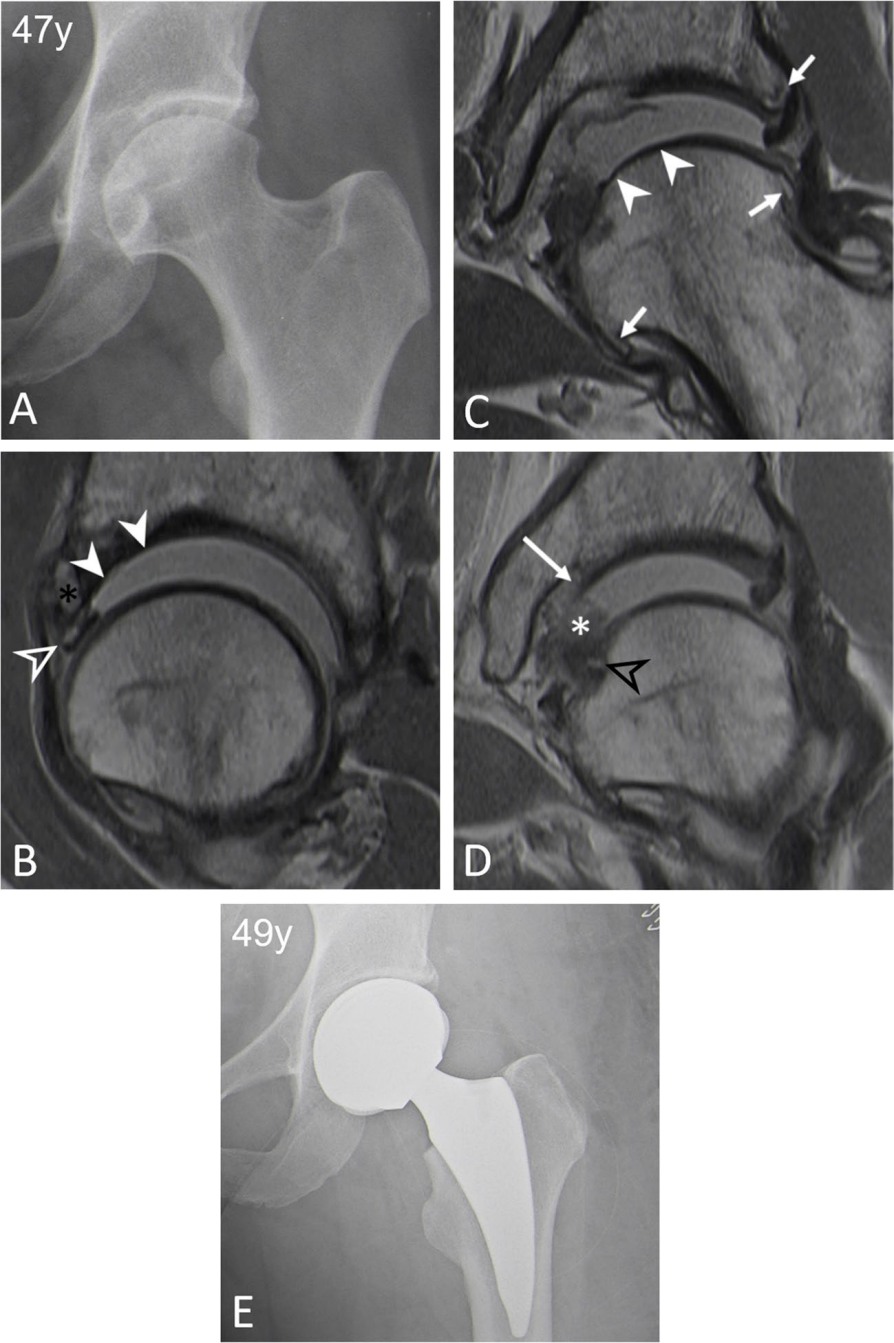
A 47-year-old woman with pincer FAI. B–D Preoperative traction MR-arthrogram with proton density-weighted turbo spin echo images showing three MRI findings independently associated with worse outcomes following FAI surgery. B The sagittal image shows an extensive cartilage defect > 2 h on the clockface (arrowheads), an associated anterior labrum lesion (empty arrowhead), and subchondral cyst formation at the acetabular rim (asterisk). C, D Consecutive coronal images demonstrate the central femoral osteophyte (black arrowhead) and a central femoral cartilage defect (arrowheads) with associated damage to the ligamentum teres (asterisk). In addition, a central acetabular osteophyte (long arrow), as well as peripheral acetabular/ femoral osteophytes (short arrows) are seen. E Postoperative radiograph following total hip replacement 2 years after the hip arthroscopy
